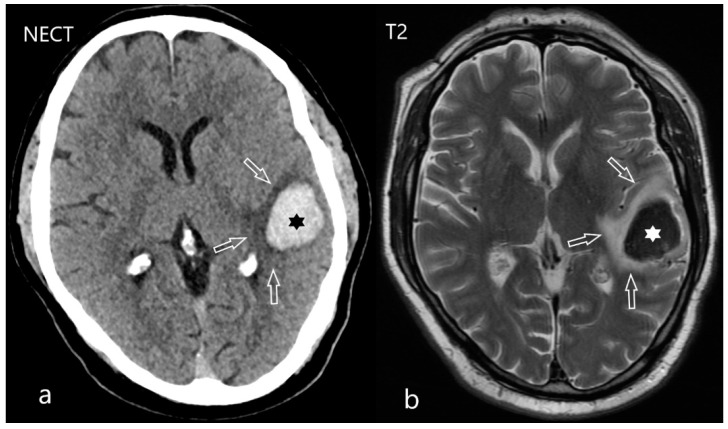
Acute intracerebral hematoma. 57-year-old male was referred with one day aphasia with acute onset. There is an acute intracerebral hematoma (star) on NECT (a) and on T2- weighted MRI (b) situated in the left temporal lobe. Perifocal vasogenic edema is also present (arrows). The source of hemorrhage was a pial arteriovenous malformation.
